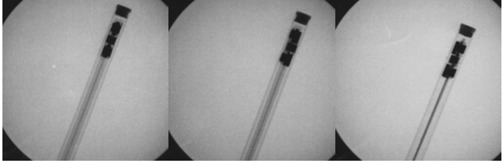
Radiographic images of the transparent detector at 0° (left), 45° (middle), and 90° (right) towards the primary radiation field.
